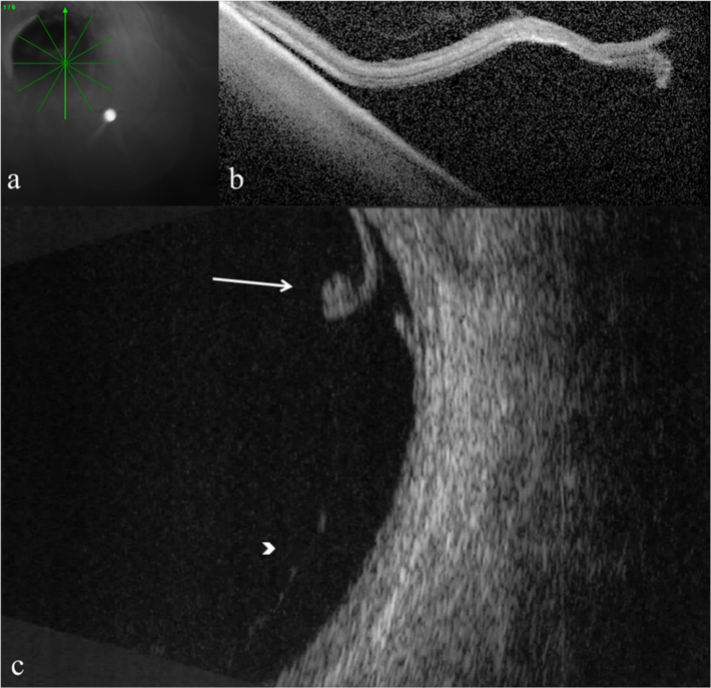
Optical coherence tomography and B-scan ultrasonography showing retinal tear and vitreo-retinal traction. Optical coherence tomography radial scan and cross-sectional images (a, b); and 10 MHz B-scan ultrasonography image (c). Arrow indicates retinal tear and arrowhead indicates vitreo-retinal traction
